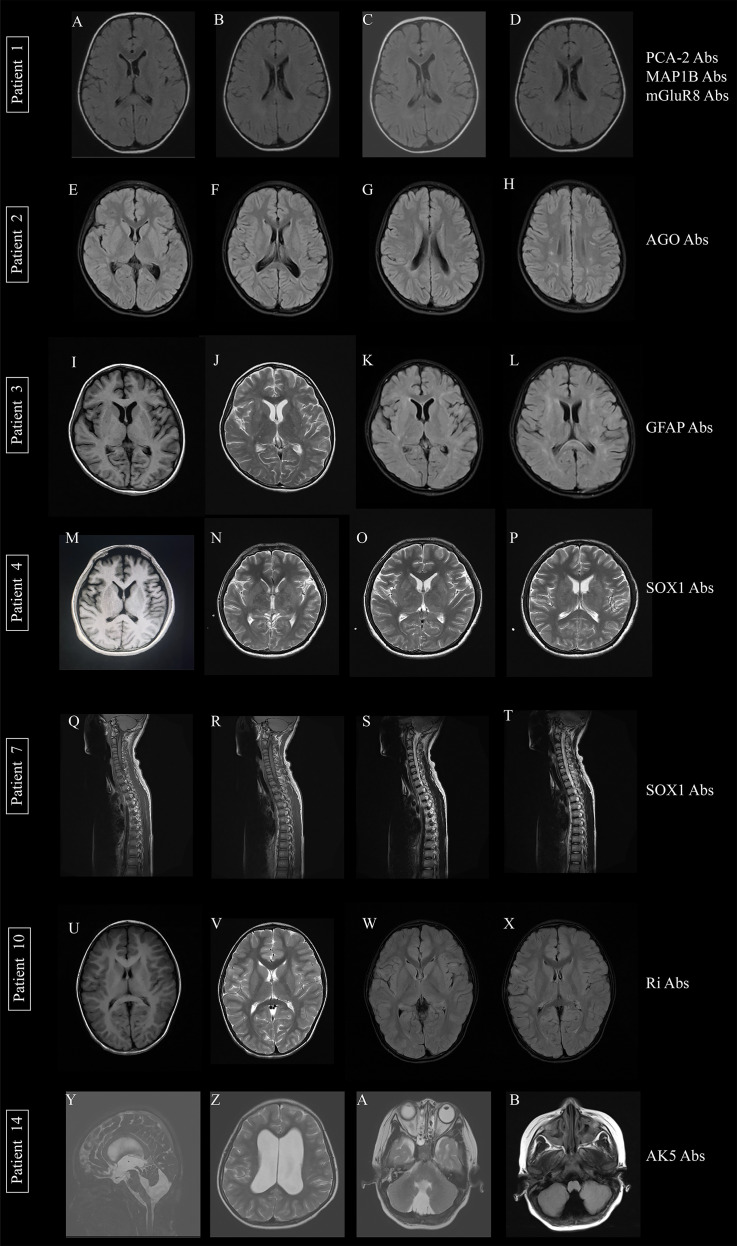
Brain MRI changes in patients with autoimmune encephalitis associated with antibodies against intracellular antigens. (A, B) Patient 1 with MAP1B Abs, mGluR8 Abs, and PCA-2 Abs, before treatment; (C, D) patient 1, after treatment; (E–H) patient 2 with AGO Abs, abnormalities around the bilateral radiative coronal and lateral ventricle triangle areas; (I–L) patient 3 with GFAP Abs, on T1WI, T2WI, and T2 FLAIR respectively; one of patients with SOX1 Abs, patient 4, showed abnormalities in the bilateral basal ganglia thalamus (M–P), while another showed myelitis possibility on T1WI and T2WI (Q–T); (U–X) the patient with Ri Abs showed long T1, T2 and T2 FLAIR signals; and (Y, Z, patient 14 A, B) the patient with AK5 Abs showed enlarged ventricle system.
